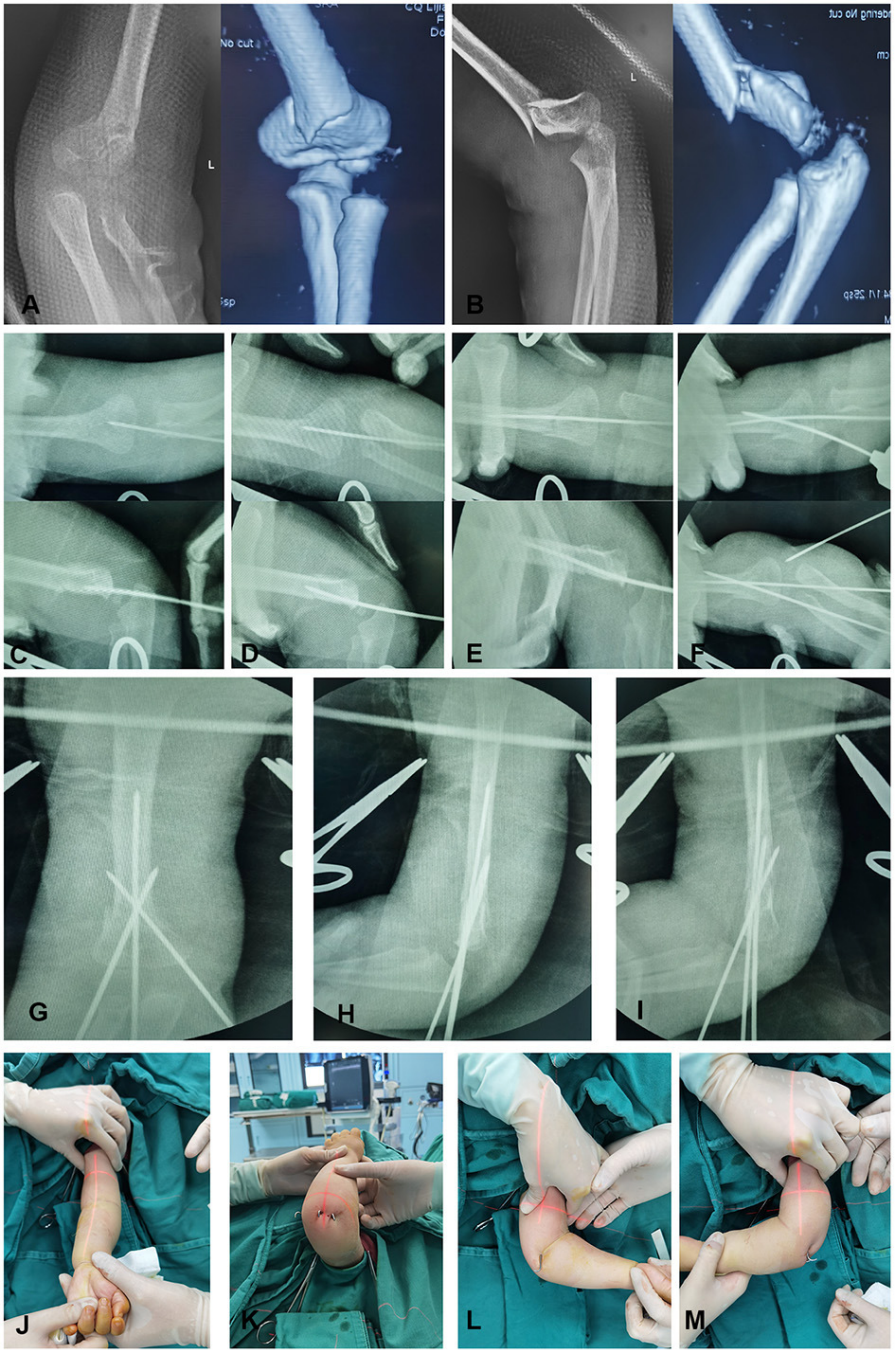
A 2-year-old girl with displaced MDJ fracture of her left elbow. (A) The anteroposterior (AP) radiograph and coronal 3D-CT image of the MDJ fracture; (B) the lateral radiograph and sagittal 3D-CT image of the fracture; (C) the AP and lateral radiographs when inserting a pin into the distal fracture end; (D) the AP and lateral radiographs where the intramedullary pin crosses the fracture line; (E) the AP and lateral radiographs when driving the pin through the fracture line and into the intramedullary canal; (F) the AP radiographs of cross-pin placement; the AP (G), lateral (H), and oblique (I) radiographs after cross-pin configuration; (J–M) the post-operative appearance of the elbow after this novel, minimally invasive procedure.
